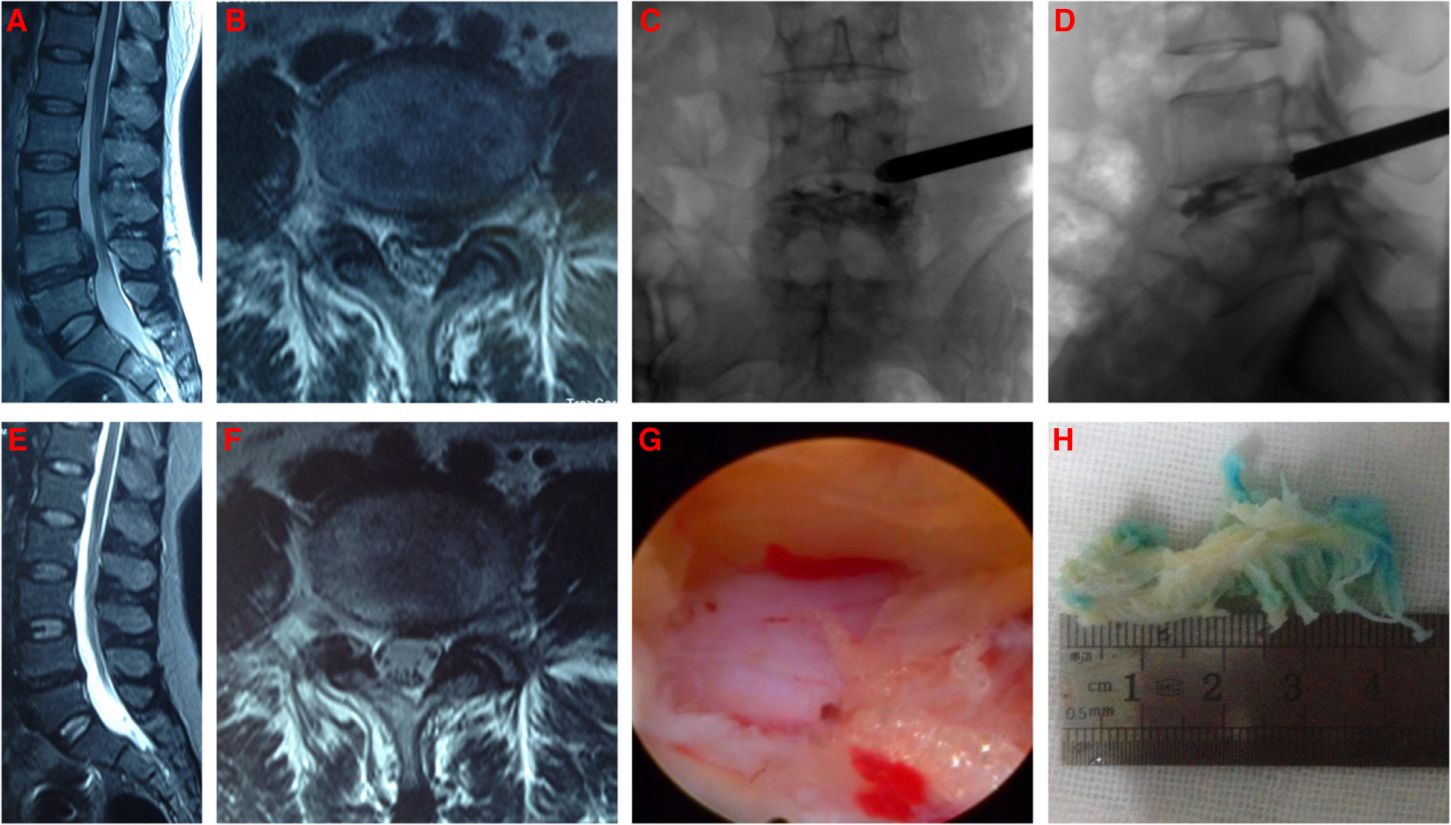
The typical PETD procedure. (A,B) Preoperative MRI of L4/5 LDH; (C,D) The working catheter of PETD; (E,F) 10 months postoperative MRI of L4/5 showing good decompression of nerve root; (G) Decompression of nerve root under endoscope; (H) Intraoperative removal of the nucleus pulposus.
